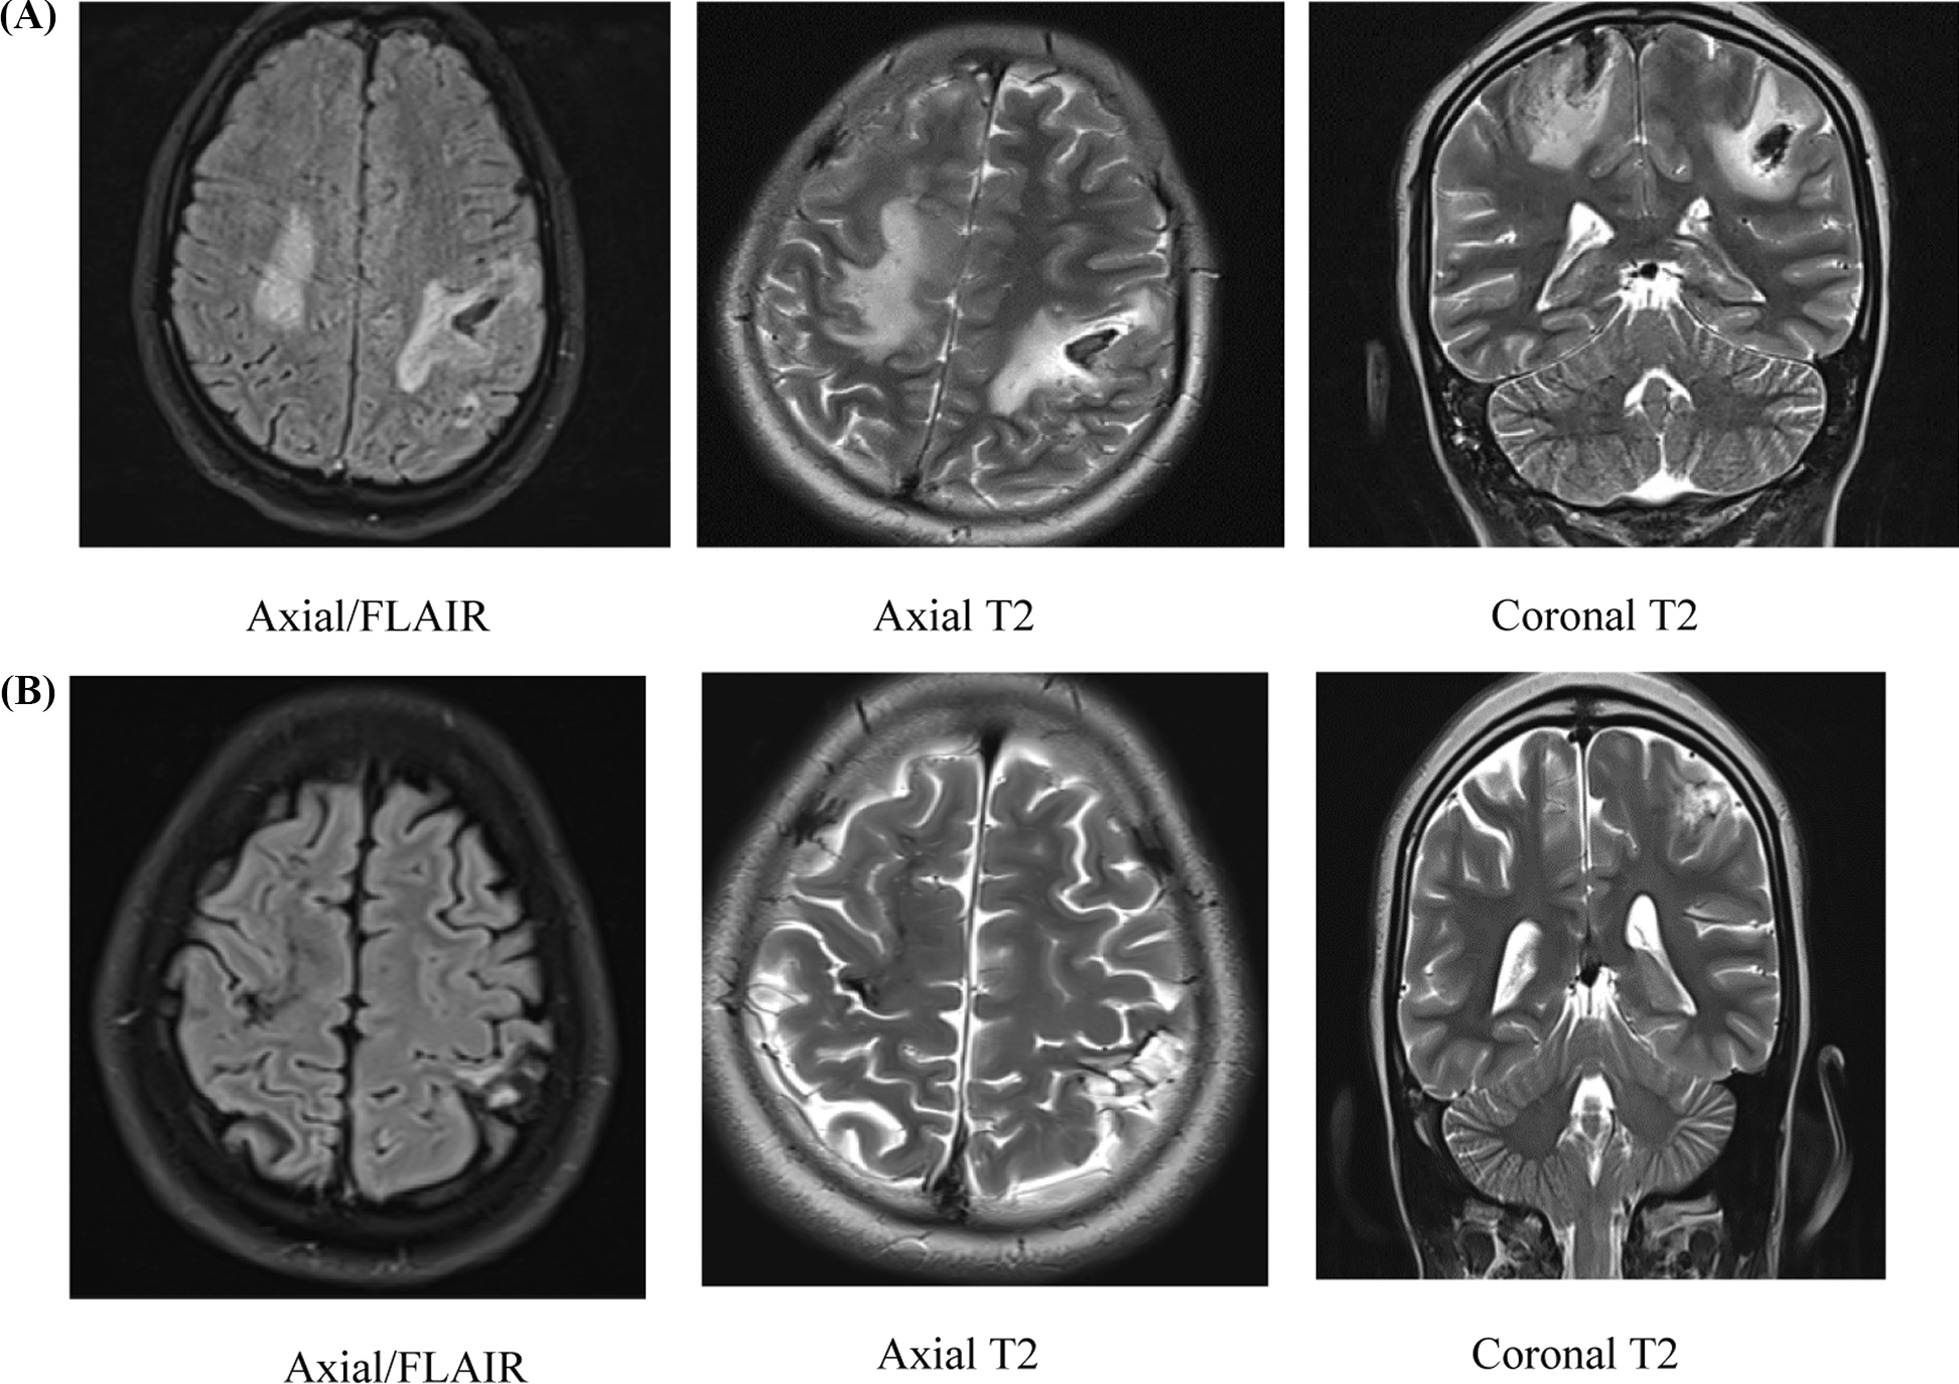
A Dark fluid and T2W sequences, axial and coronal images showing dilated tortuous tubular and serpentine structures of signal void appearance in T2/FLAIR sequences in the bilateral fronto-parietal cerebral lobes with surrounding notable vasogenic edema. B Dark fluid and T2W sequences, axial and coronal images: Reduced tortuous tubular and serpentine structures of signal void appearance in T2/FLAIR sequences are noted in the bilateral parietal cerebral lobes. In Axial T2 and Coronal T2, complete resolution of right parietal vasogenic edema and reduced surrounding vasogenic edema seen
